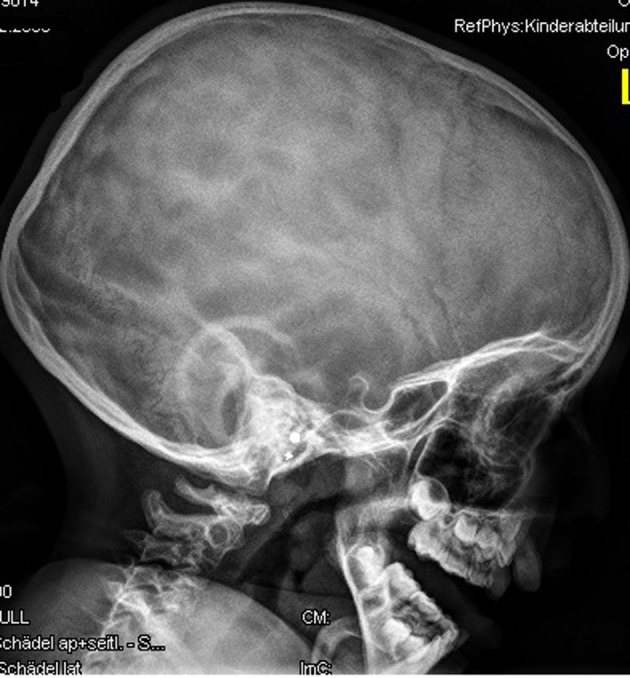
Lateral skull radiograph showed obtuse angle of the mandible, wormian bones, hypoplasia of the zygoma, mandible and maxilla respectively.
